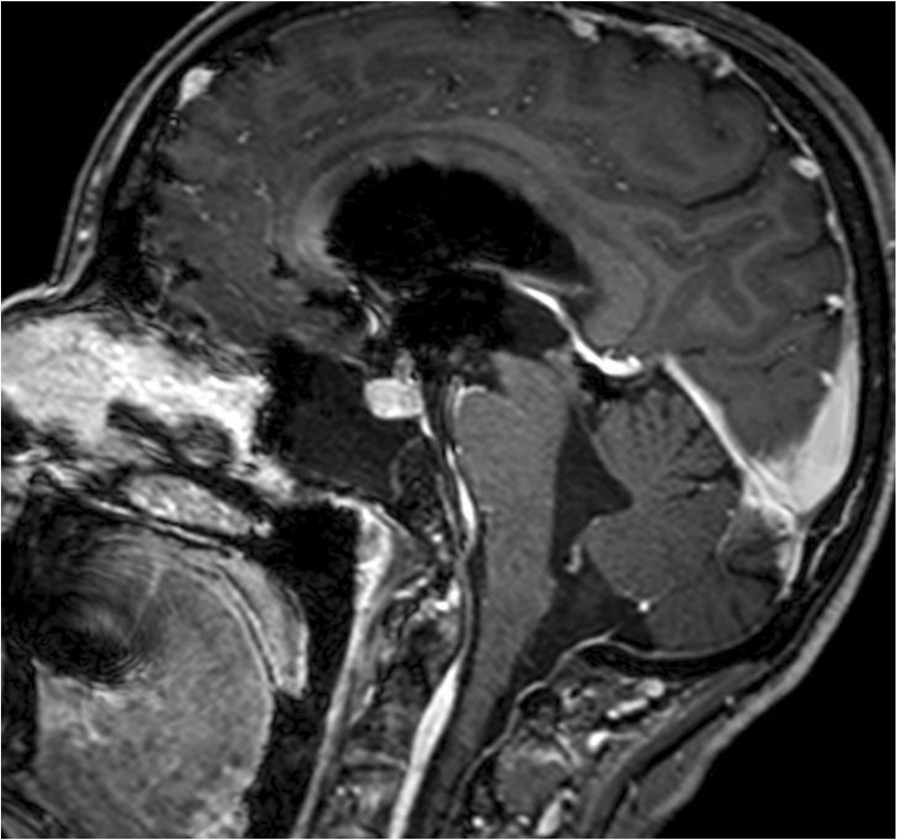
T1-weighted sagittal images showing an osteolytic lesion of the clivus with no contrast enhancement.
